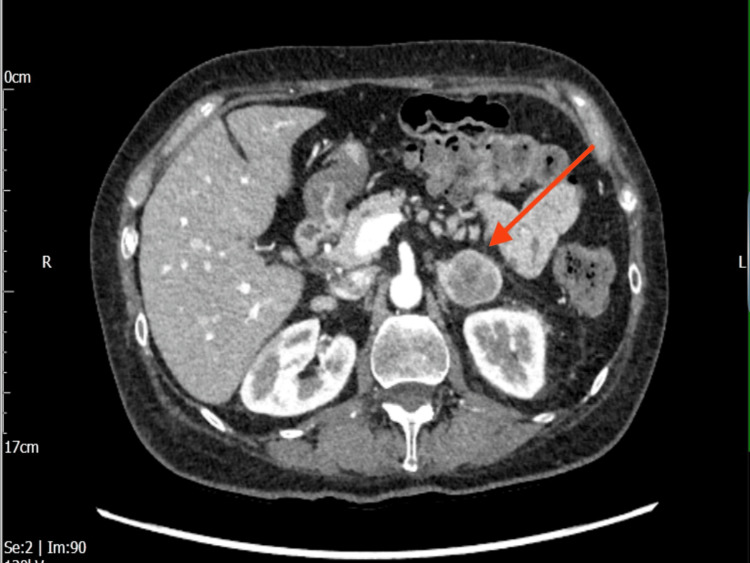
CT biphasic abdomen and pelvis with contrast demonstrating a large, solid, heterogeneous left adrenal nodule measuring 3.4 cm × 2.8 cm (red arrow) CT: computed tomography
